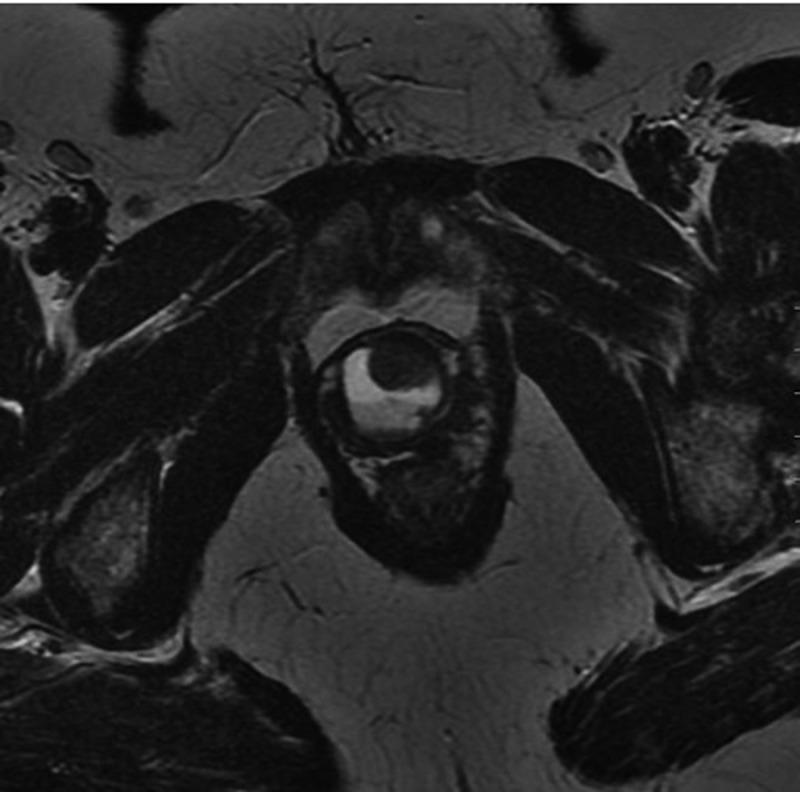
Axial T2-weighted MRI image of a 2.3-cm saddlebag UD.
